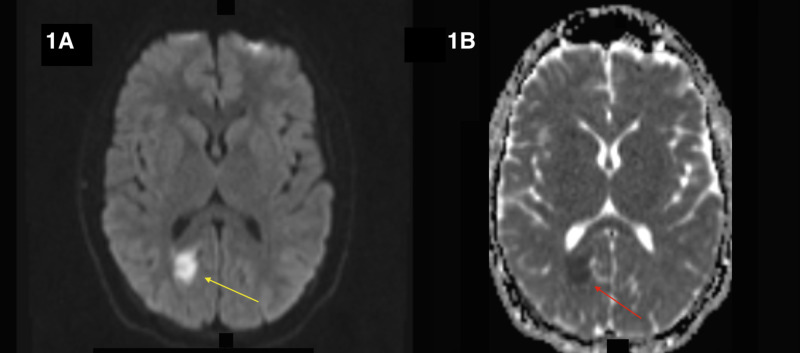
(A) Diffusion-weighted imaging (DWI) shows hyperintensity of banks of parieto-occipital sulcus (yellow arrow). (B) Matching hypointensity on gradient echo (GRE) imaging (red arrow).
